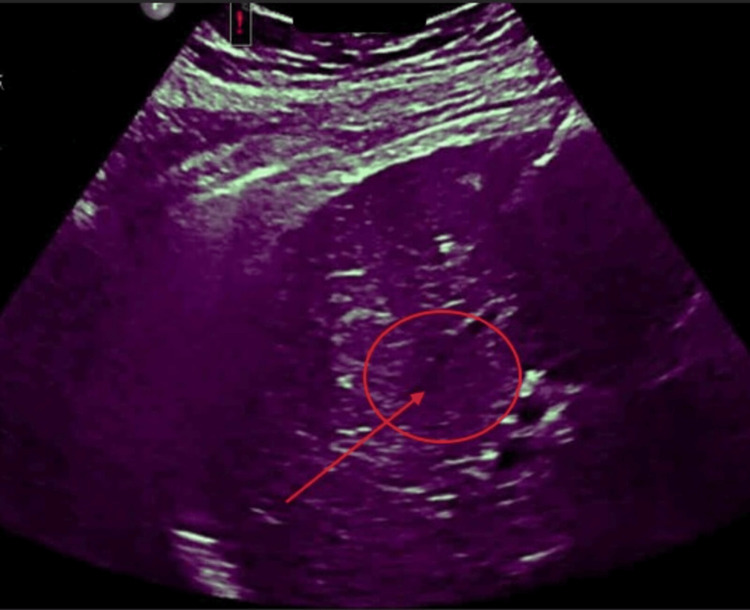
Post-ablation color Doppler ultrasound demonstrating the ablation zone. Color Doppler imaging performed immediately following microwave ablation shows the expected non-enhancing ablation zone encompassing the treated lesion in hepatic segments 7 and 8 (red annotations). A small perihepatic hematoma was noted, which remained stable on serial imaging and did not require intervention.
